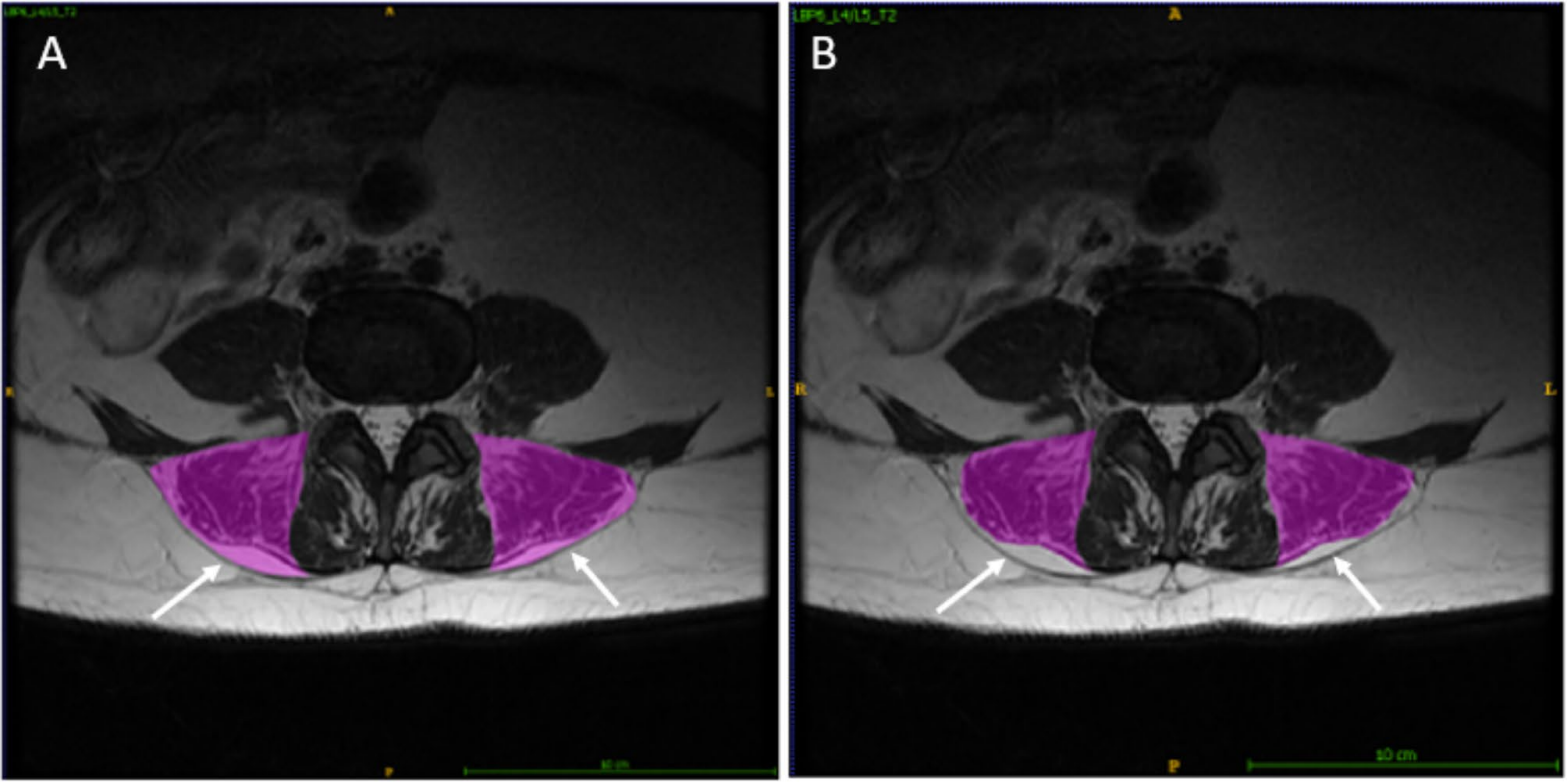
T2-weighted images at the L4/L5 level in a female with LBP. (A) includes the epimuscular fat in the CSA measurement. (B) excludes the epimuscular fat in the CSA measurement
